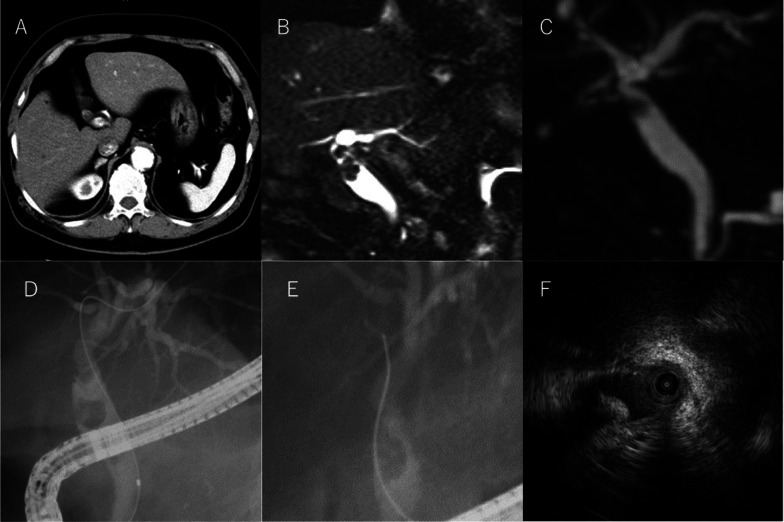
Preoperative findings of choledocholithiasis in "Case 2". A CT image shows a mass located in the upper bile duct. B, C MRCP imaging shows a mass located in the upper bile duct. D, E ERCP image shows a filling defect in the upper bile duct, which suggests bile duct cancer. F Intraductal ultrasonography image reveals an intraductal tumor
